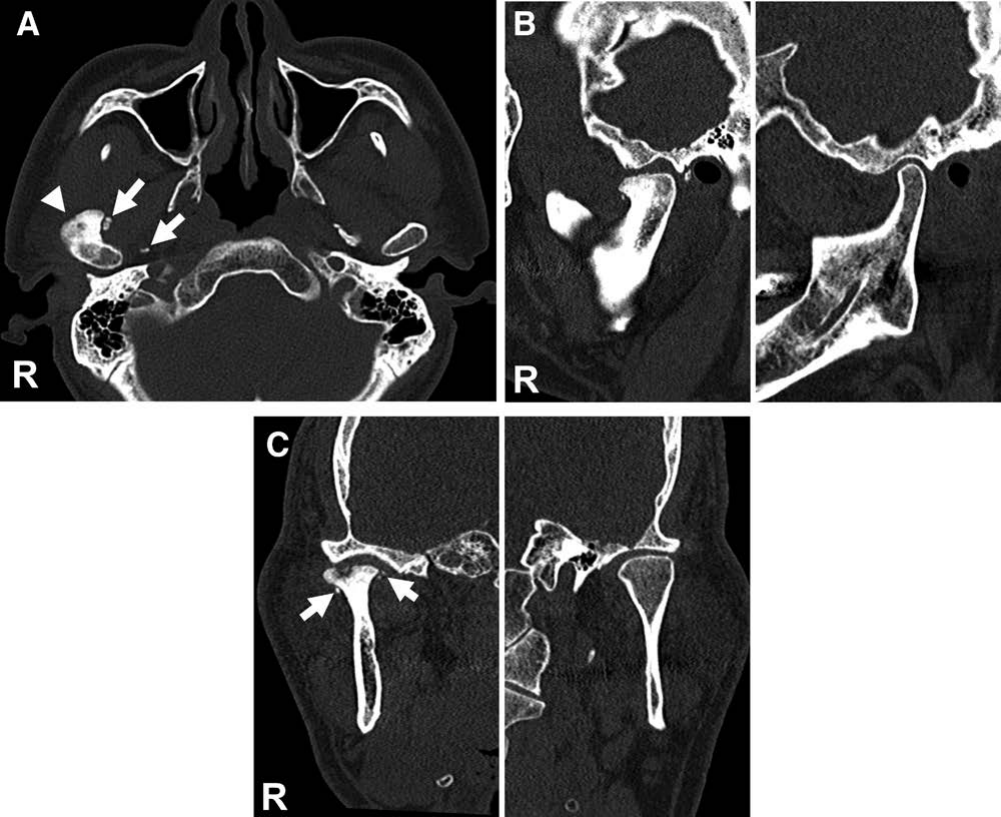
Preoperative computed tomography (CT). (A) Axial view, showing a radiopaque mass fused to anterolateral surface of the right condylar head (arrowhead) with several calcified masses (arrows). (B) Oblique sagittal view of the temporomandibular joint (TMJ), showing the right condyle displaced from the articular fossa. (C) Coronal view of the TMJ, displaying osteoarthritic changes along with several calcified fragments (arrows) on the medial and lateral aspects of the right condyle.
